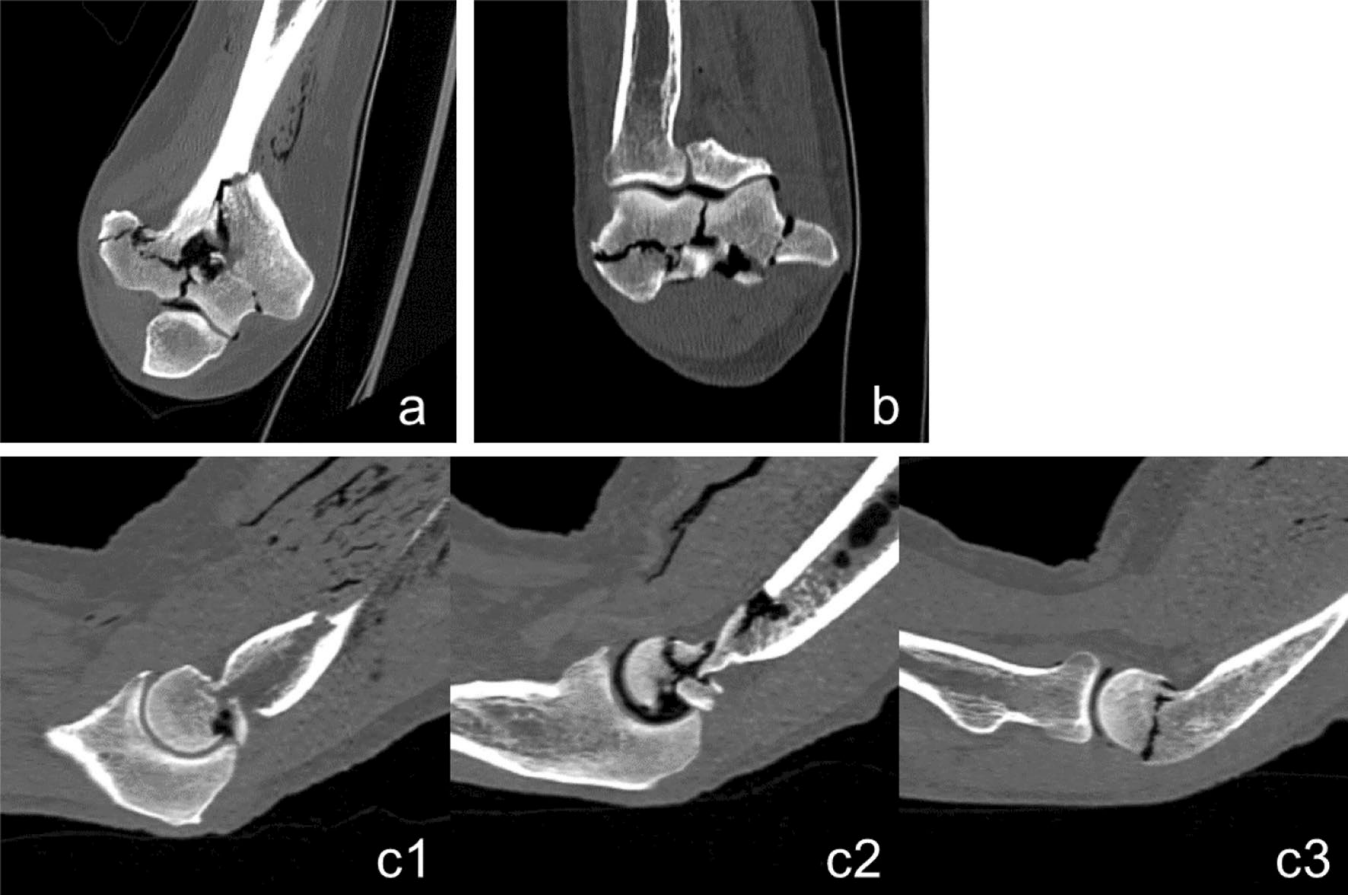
Exemplary CT cross sections of a typical humerus fracture pattern (AO-classification 13C3.2) a coronal plane, b axial plane, c1–c3 sagittal plane
